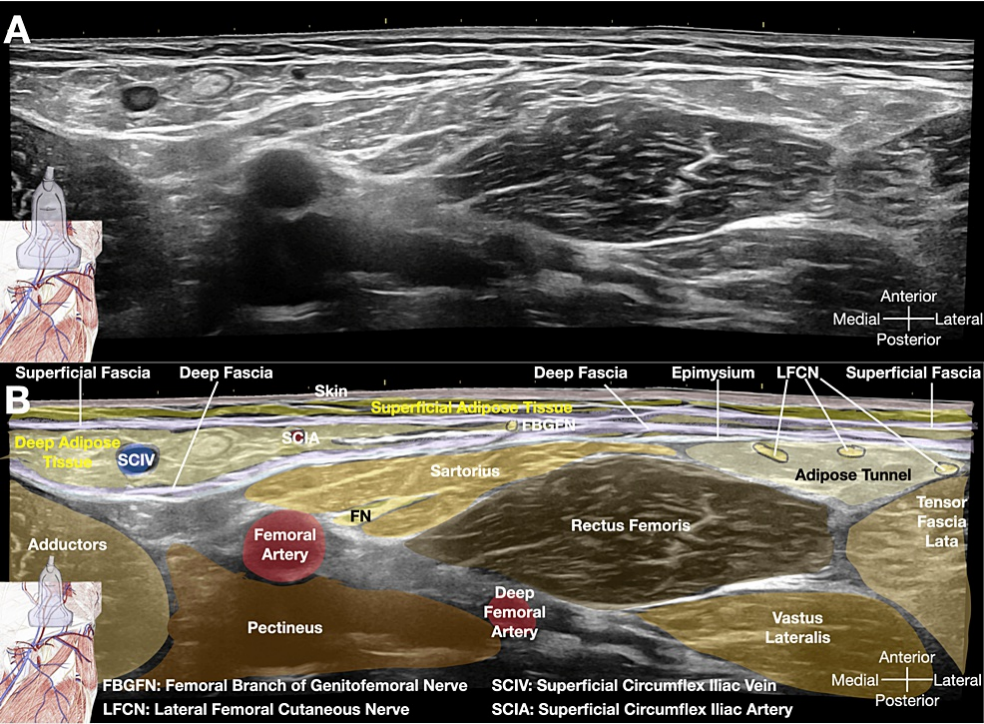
Sonoanatomy of the structures caudal to the inguinal ligament corresponding to the five dots in Figure 1 This figure demonstrates the sonoanatomy of the structures located caudal (inferior) to the inguinal ligament, corresponding to the five dots shown in Figure 1. Sub-figure A presents the original ultrasound image, while Sub-figure B provides a color-coded mapping of the structures, along with detailed labels for the anatomical features visible in Sub-figure A. Image credit: Dr. Lam K
